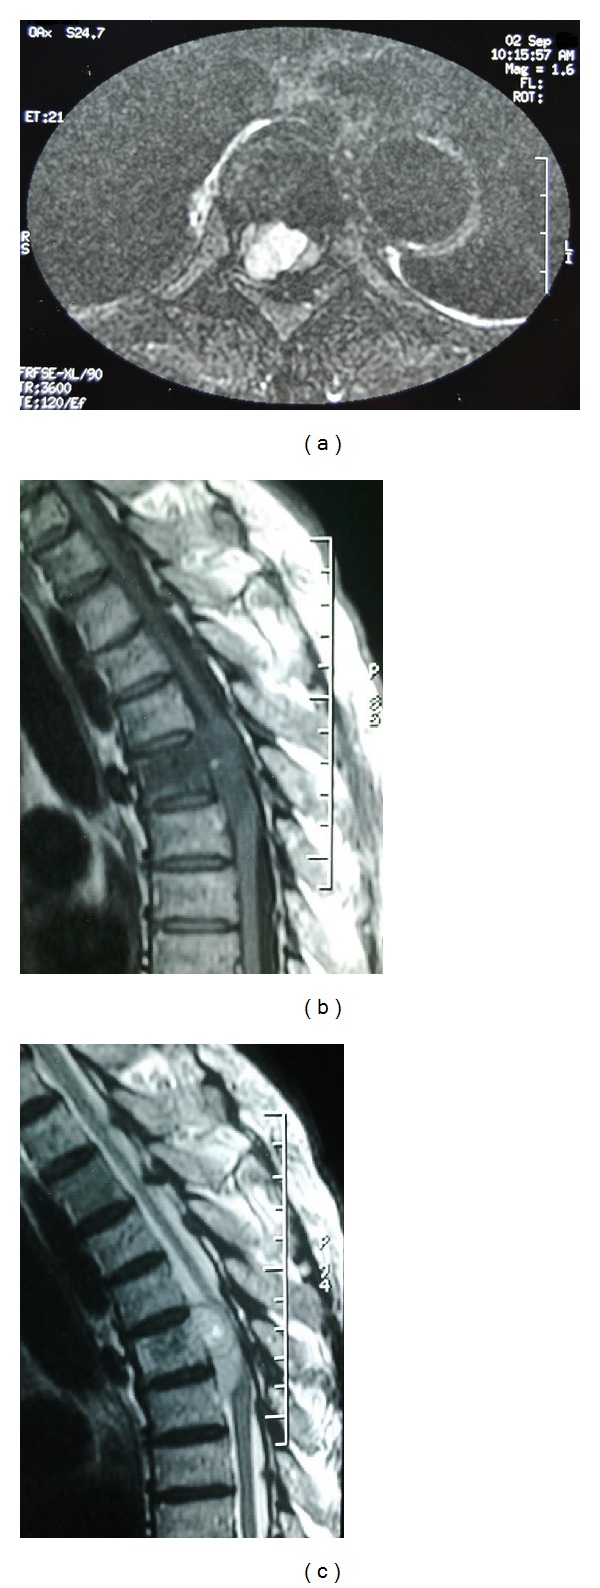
Preoperative axial (a) and sagittal (b) T1-weighted and sagittal (c) T2-weighted MR images through the thoracic spine showing a T1 hypointense, T2 hyperintense, extradural process at T5 level with important spinal cord compression. Note the T5 vertebral body signal changes.
